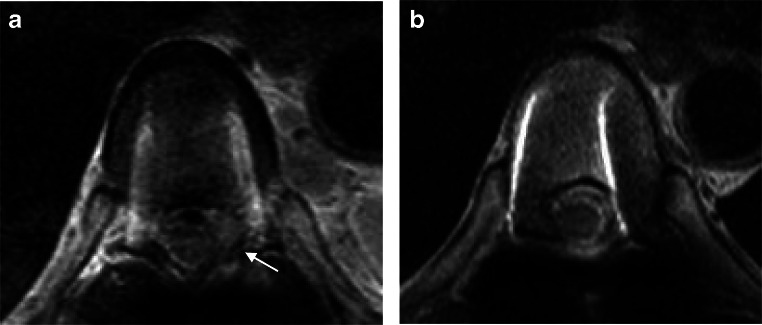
Postoperative T2-weighted magnetic resonance images (axial view). (a) One month after surgery. The spinal cord appeared sufficiently decompressed. Partially resected tumor in epidural space and the remaining tumor on the lateral side (*white arrows*) were confirmed. (b) Six months after surgery. Both epidural and lateral vertebral masses were not observed
